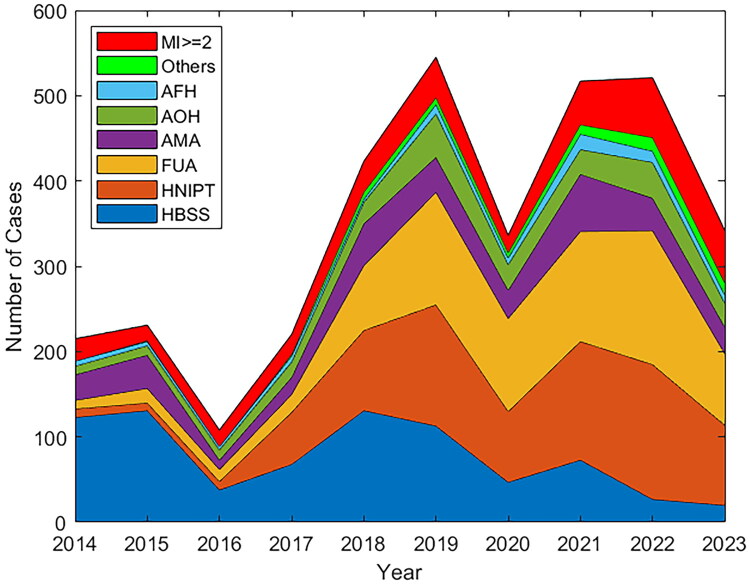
Trend of prenatal diagnostic indications in Heilongjiang Province (2014–2023) is displayed using stacked area plot. HBSS: high-risk biochemical serological screening; HNIPT: high-risk NIPT; FUA: fetal ultrasound abnormalities; AMA: advanced maternal age; AOH: adverse obstetric history; AFH: adverse family history; MI: multiple indications.
